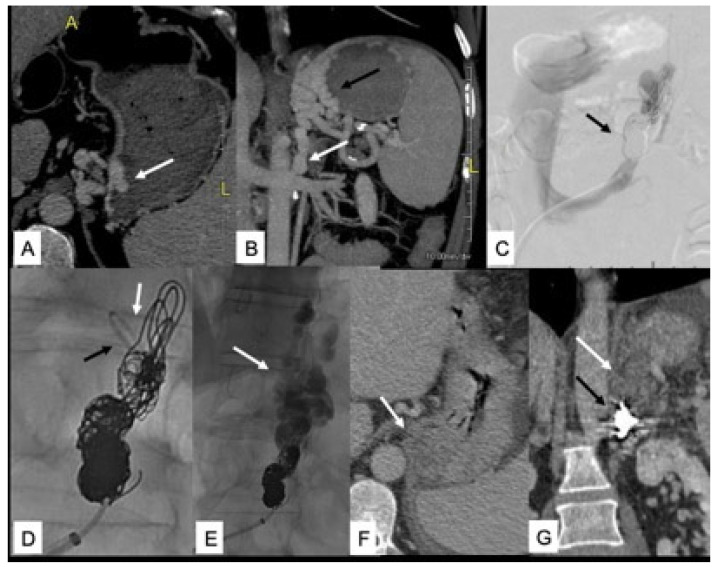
(A,B) Pre-procedural CT axial and coronal reformats show intra- and extramural gastric varices (GV) (white arrow in (A) and black arrow in (B)) and GRS (white arrow in (B,C)). Coil embolization of GRS (black arrow) with persistent shunt patency. (D) Angiographic catheter distal to coils (black arrow) and coaxial microcatheter looped backward for NBCA injection (white arrow). (E) Extensive filling of GV with gelfoam and contrast media (white arrow). (F,G) Post-procedural CT shows complete thrombosis of GV and GRS (white arrow) and coils in the GRS (black arrow).
